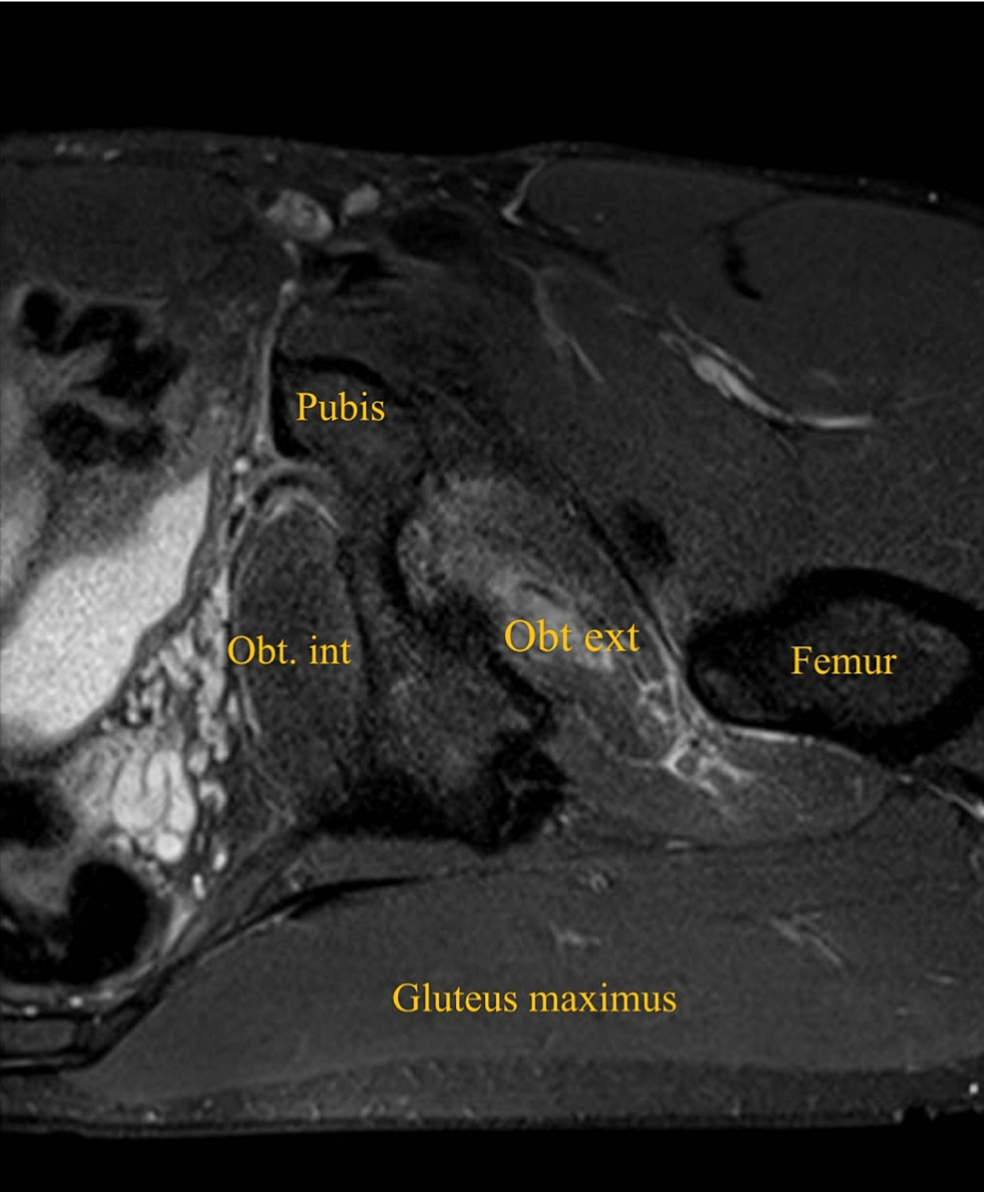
Axial short-tau inversion recovery (STIR) MRI of the left thigh showing left obturator externus tear Obt. ext: obturator externus; Obt. int: obturator internus
